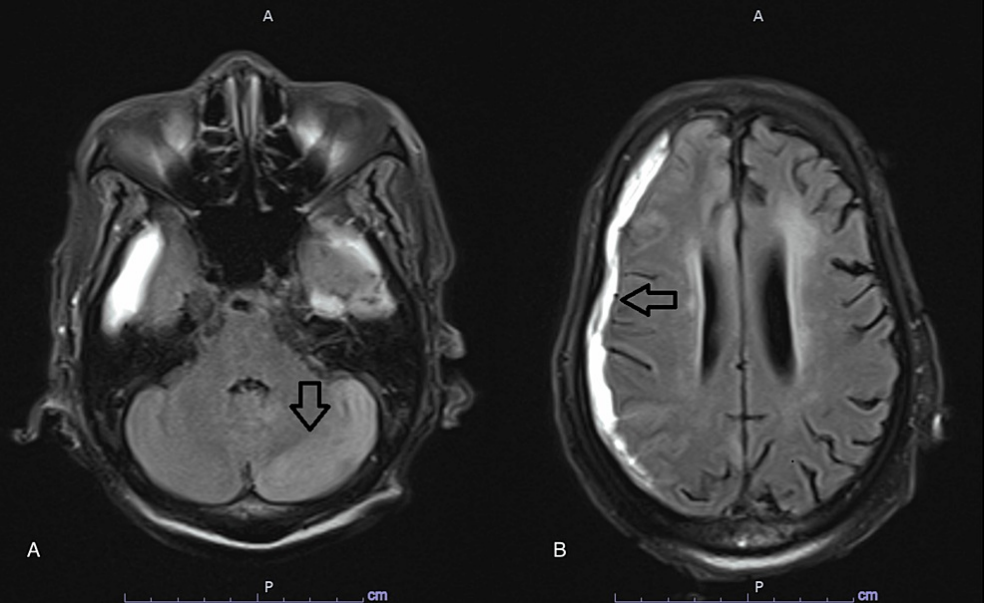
MRI FLAIR sequence Magnetic resonance imaging (MRI) brain fluid-attenuated inversion recovery (FLAIR) sequence showing left-sided cerebellar hyperintensity (arrow in A) and right-sided subdural hemorrhage (arrow in B)
